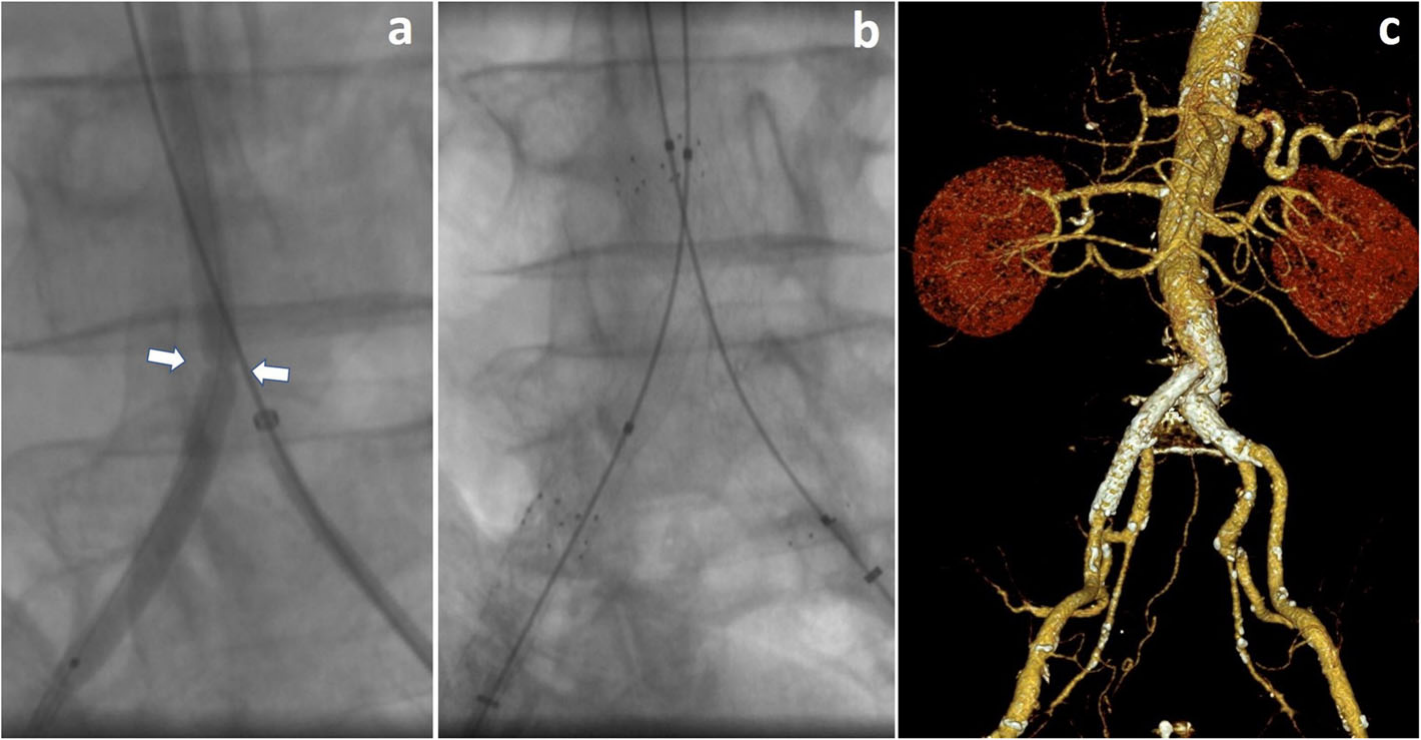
a Dilation with a low-profile ballon. Note the resistance to expansion in proximity to the passage created by the GoBack needle (arrows). b Deployment of aortoiliac kissing stents. c CT-scan follow up at 1 month
